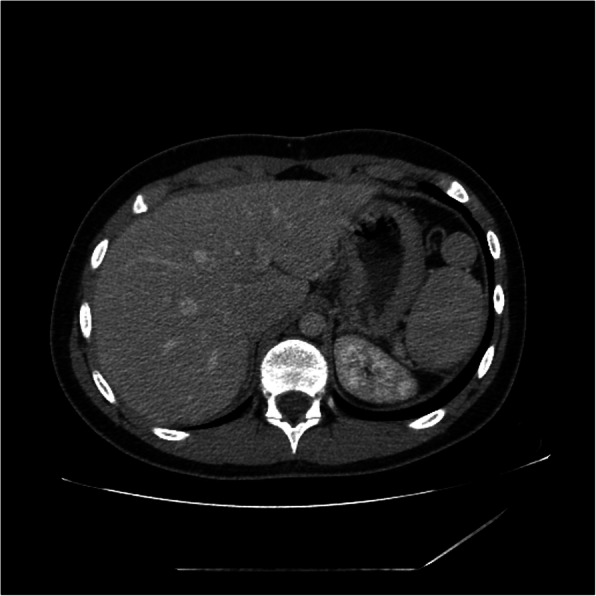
CT-scan 7 months after the surgical procedure showing the grown intraperitoneal accessory spleen in the splenic cavity and the retroperitoneal accessory spleen
